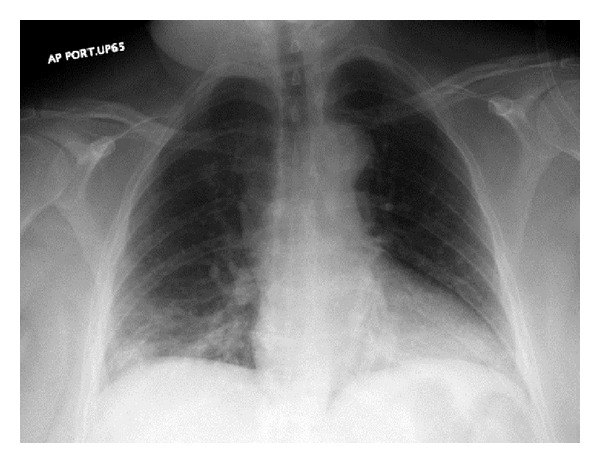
Normal chest radiograph performed prior to abdominoplasty showing no evidence of a diaphragmatic hernia.
